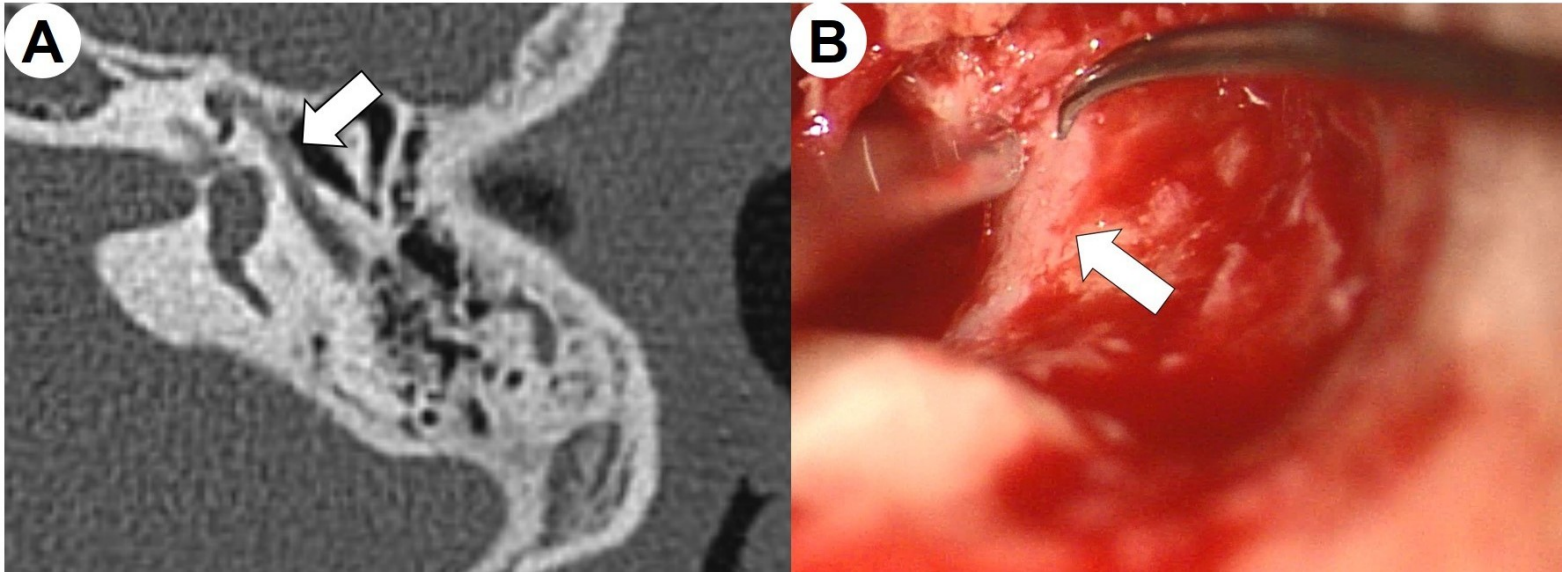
Preoperative temporal bone computed tomography scan (A) and intraoperative surgical inspection (B) showing bony canal dehiscence in the tympanic segment of the facial nerve (arrow).
